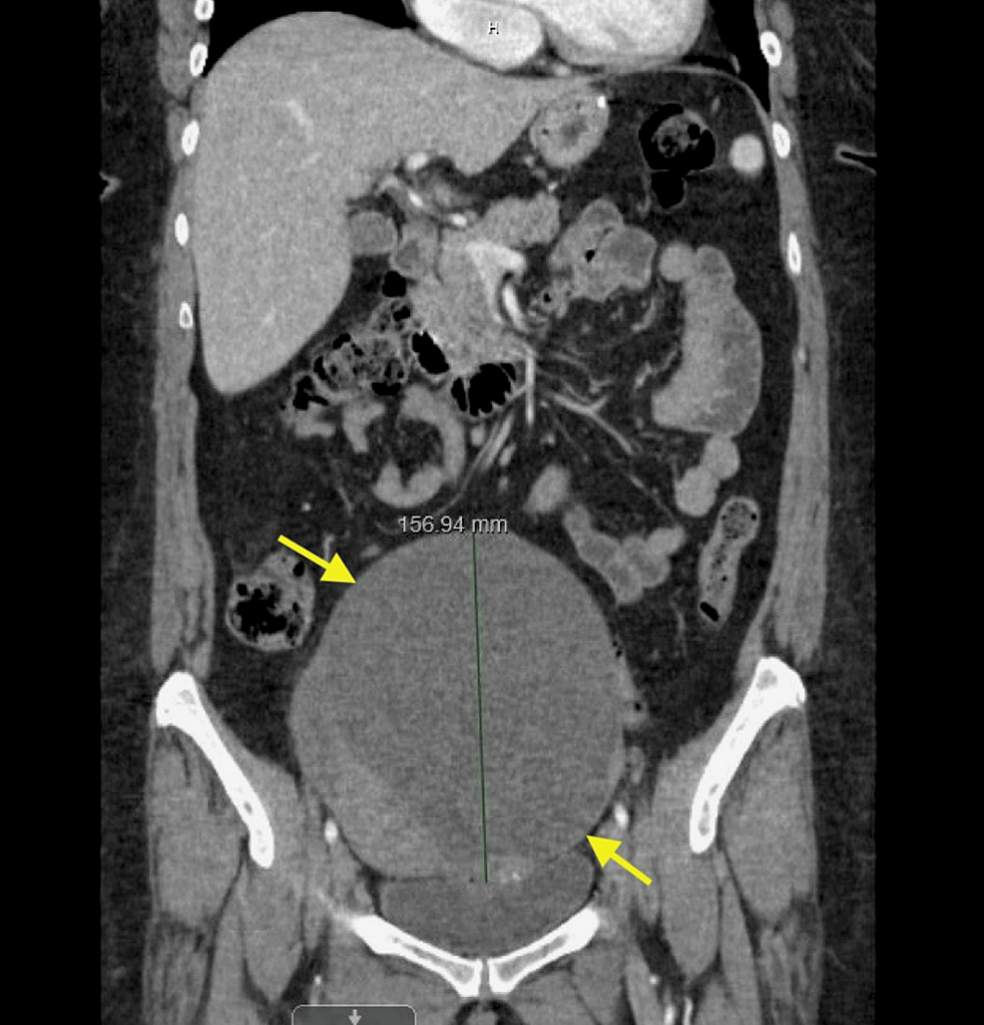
Coronal contrast-enhanced abdominal and pelvic CT revealing a large cystic pelvic mass (yellow arrows).
